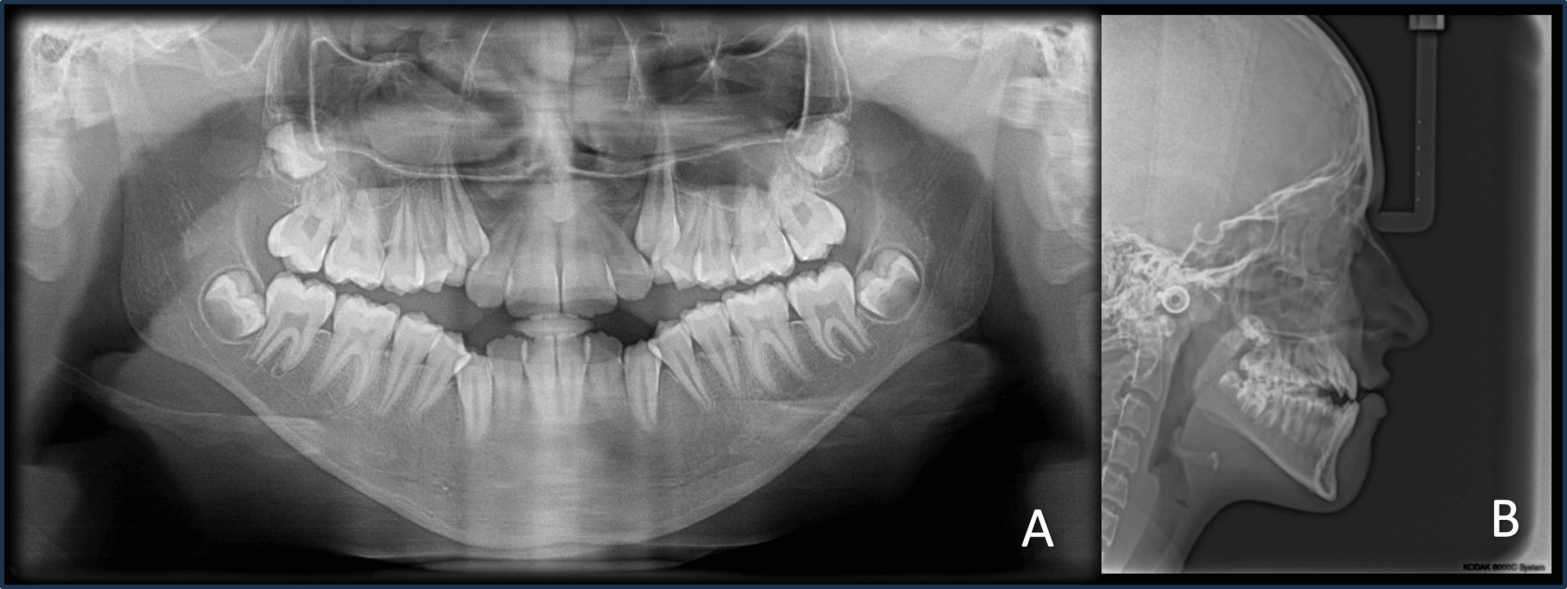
Pre-treatment radiographs A) Panoramic view; B) Lateral cephalogram
